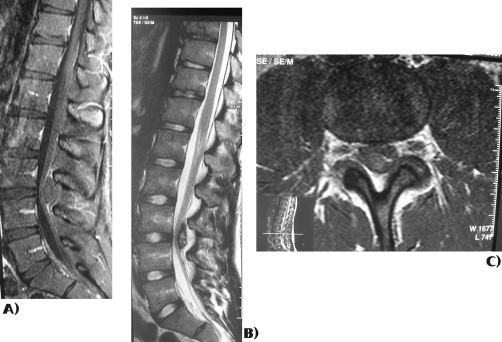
MRI scans show the epidural hematoma at the level of L3-L4.
